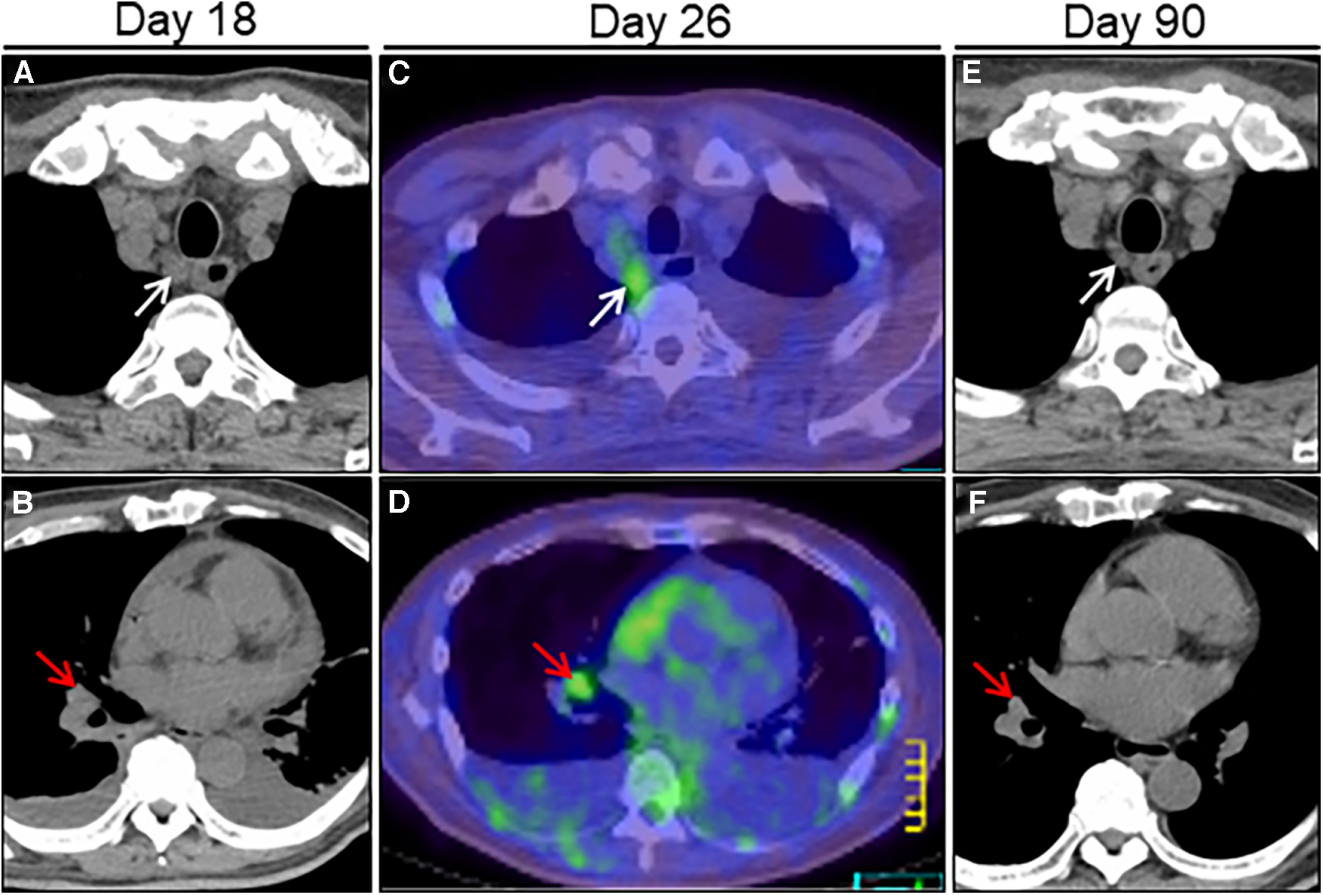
Treatment effect on lymphadenopathy on chest computed tomography (CT) imaging. Chest CT on day 18 after admission reveals right paraesophageal (white arrow) and hilar (red arrow) slight lymphadenopathies (A, B). FDG/PET-CT on day 26 reveals slight hypermetabolic activities in the same ones (C, D). Post-treatment follow-up CT on day 90 reveals a significant improvement in size of the affected lymphadenopathies (E, F). FDG/PET, positron emission tomography with 2-[18F]-fluoro-2-deoxy-D-glucose.
